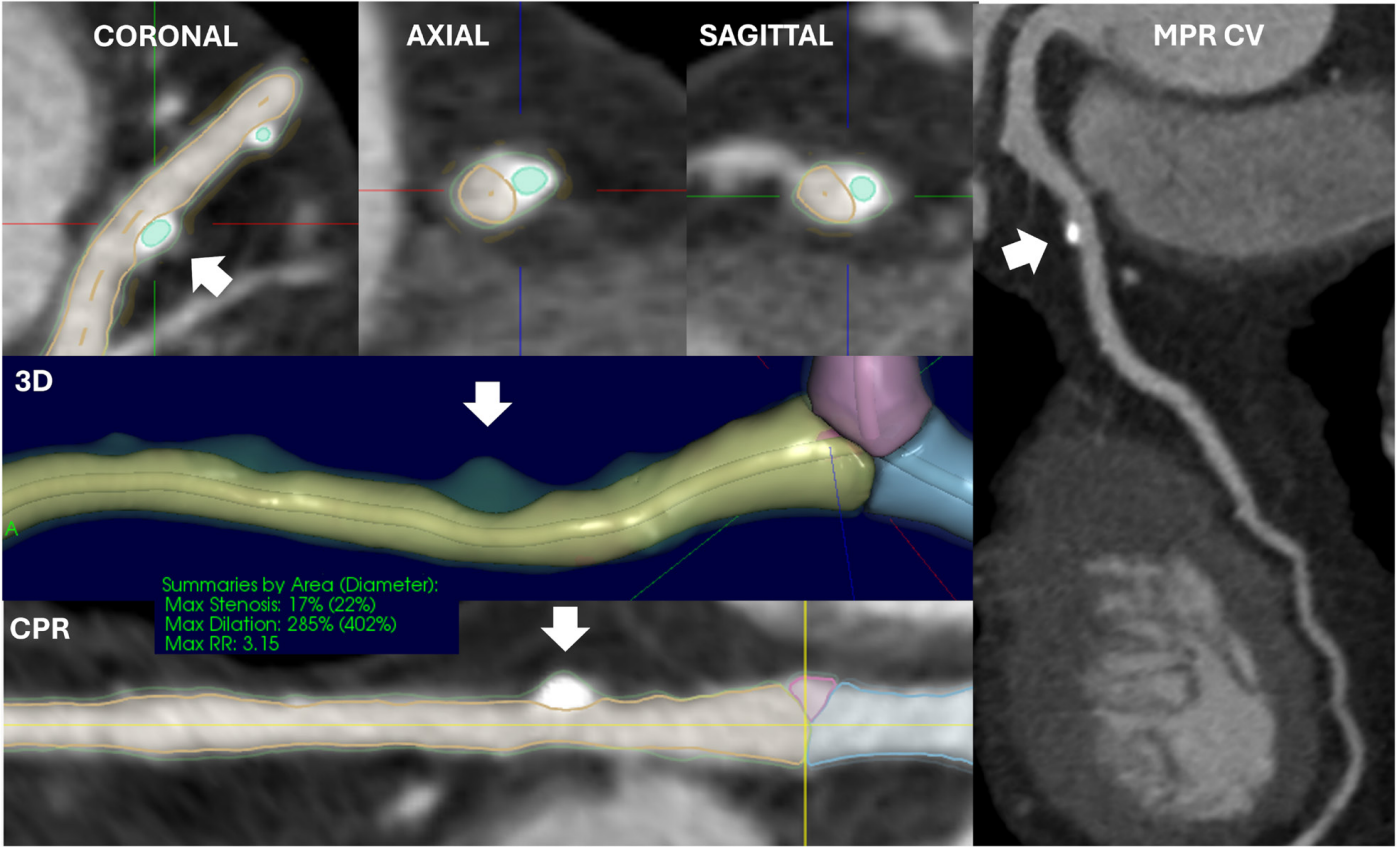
Coronary computed tomography angiography images of a 68-year-old male patient presenting with chest pain to the emergency department and multiple risk factors for coronary artery disease. Images show a calcified atherosclerotic plaque in the proximal left anterior descending (LAD) with positive remodeling with mild stenosis, ≈30% (white arrow). Coronary plaque analysis was performed with a semiautomatic software (vascuCAP; Elucid Bioimaging, Wenham, MA). Software analysis provides coronal, axial, and sagittal views, as well as 3-dimensional (3D) and curved planar reformation (CPR) straightened views. Software output includes remodeling ratio (RR), percent dilation, and percent stenosis, which in this case were 3.15, 285%, and 17%, respectively. Curved multiplanar reformation of the LAD is shown on the right. Max indicates maximum; and MPR CV, multiplanar reformation curved view.
